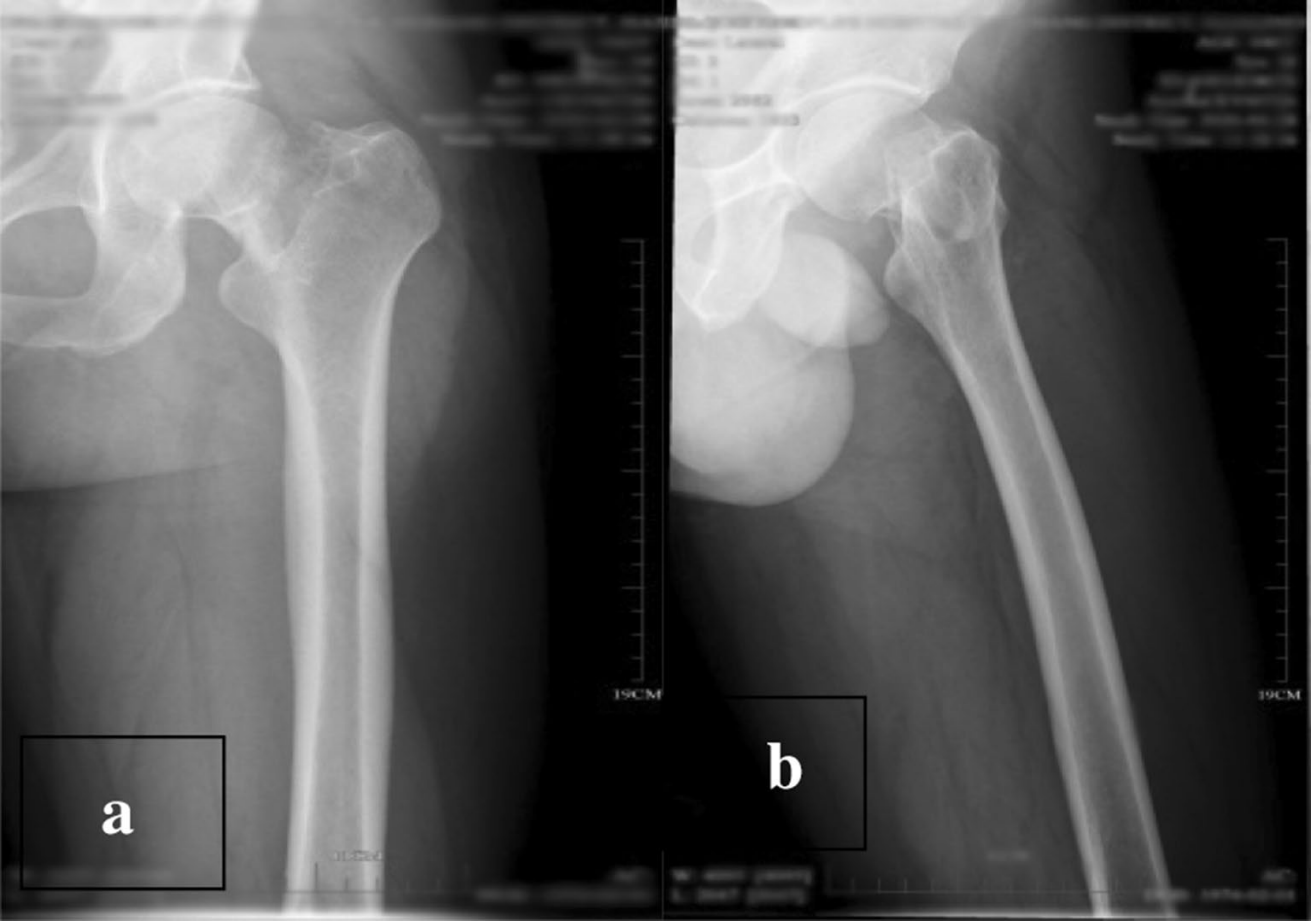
(a, b) The positive side radiographs of the injured side of the patient at the time of admission (*Note*: The patient was a 47-year-old male. He was admitted to the hospital for 2 h due to a high fall injury).
